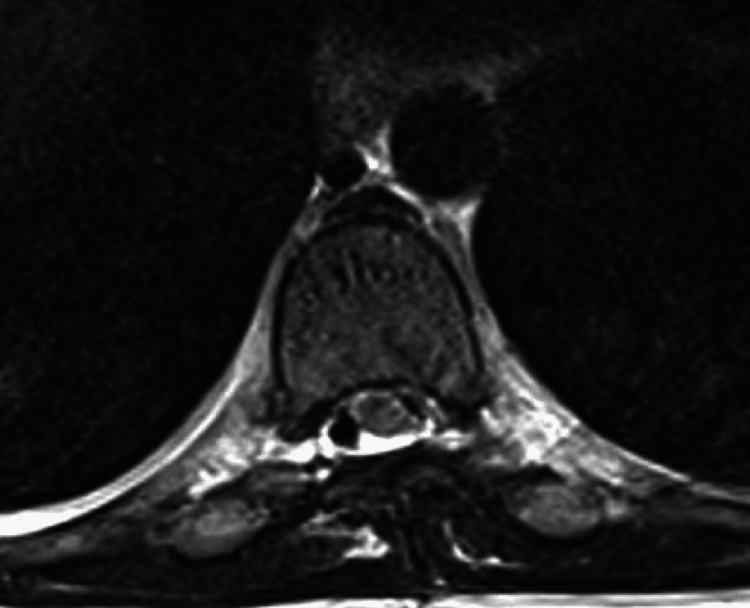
Postoperative axial T2-weighted MRI at the T8 level confirming resolution of the spinal cord herniation without additional or persistent dural defects or epidural fluid collections.
